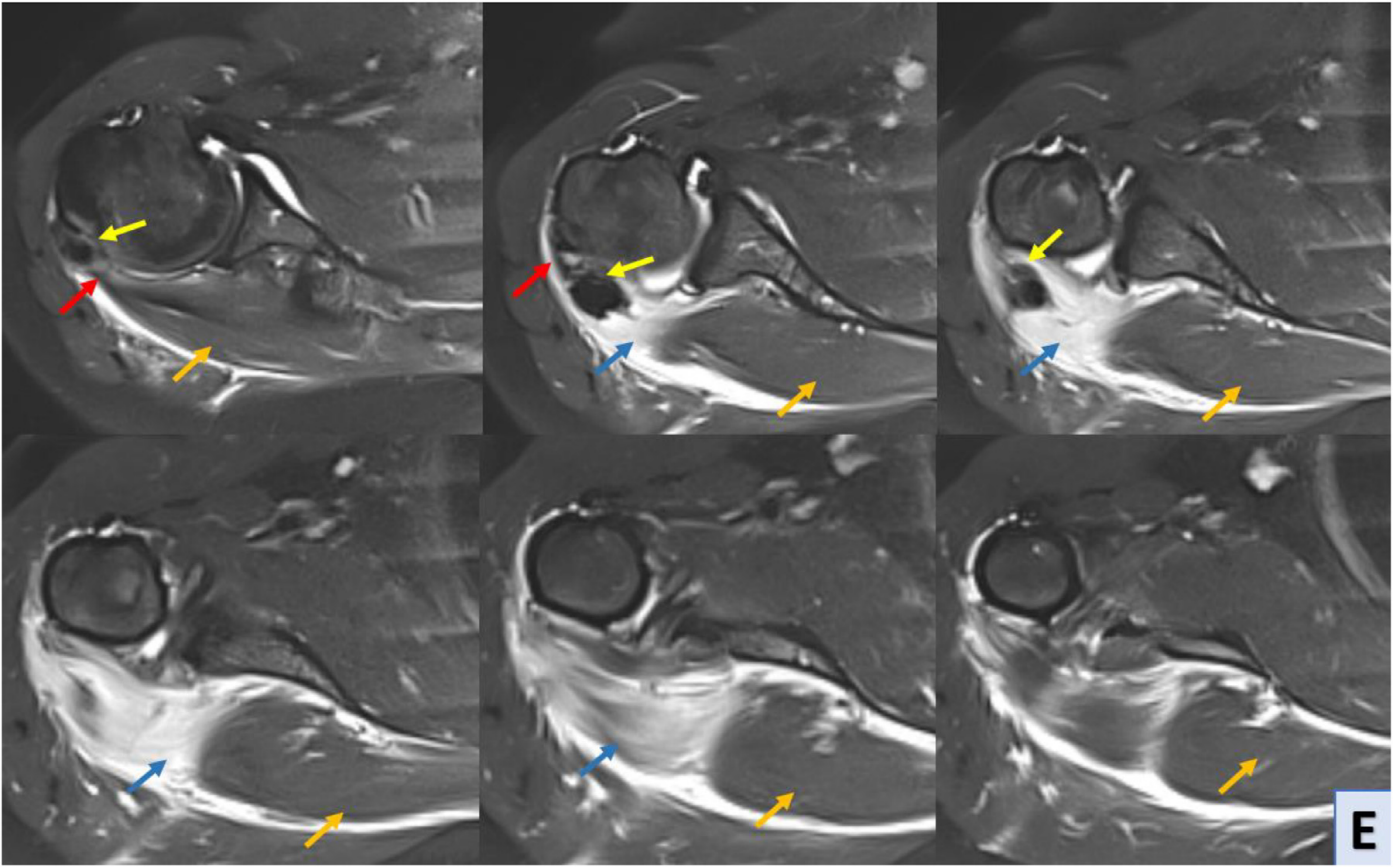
Shoulder MRI with sagittal T1 sequence (C), sagittal Blade DP FS (D) and axial Blade DP FS (E) sequences; MRI reveals asignal calcified images (yellow arrow) within the distal infraspinatus tendon (red arrow), and deeply and distally in the belly of the infraspinatus muscle. This intramuscular calcification is associated with hypersignal muscle edema (blue arrow), contrasting with the normal infraspinatus muscle (orange arrow).
